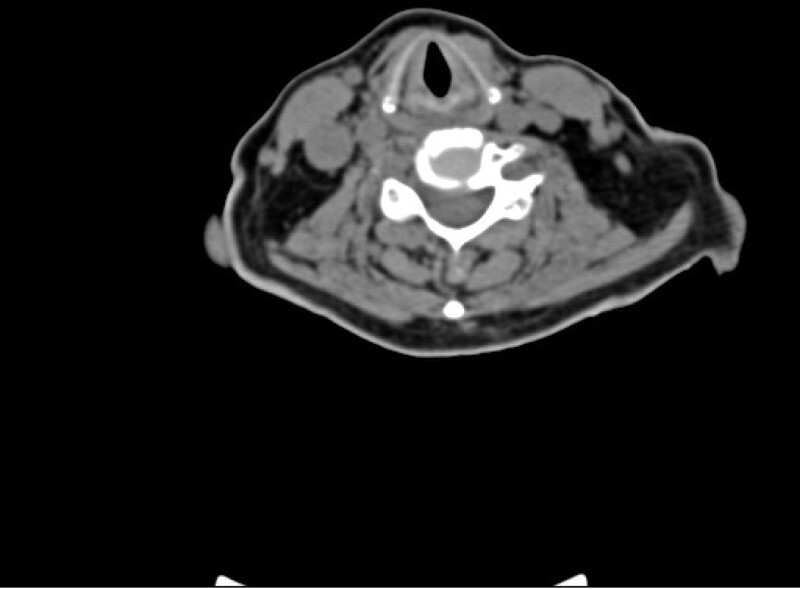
CT after extubation: CT scan of the neck after tracheal catheter extraction showed that soft tissue swelling around the glottis was reduced. CT = computerized tomography.
